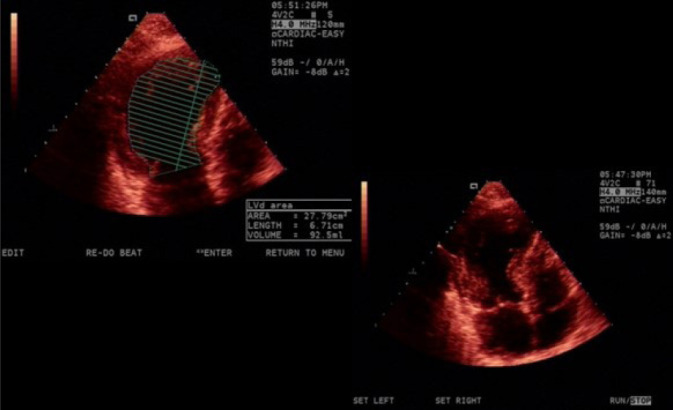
Echocardiogram (diastole and systole) from February 6, 2013 showing the typical Takotsubo left ventricular abnormality.
